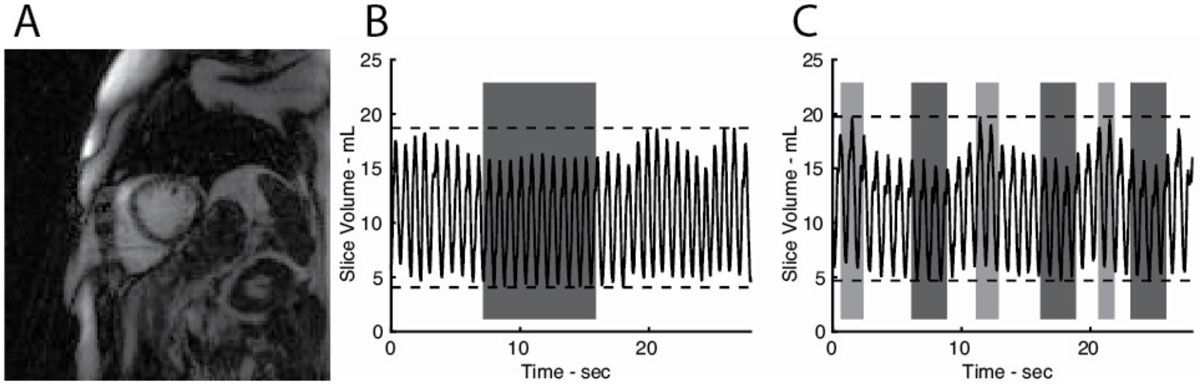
Effect of Respiratory Motion on MRI-derived Hemodynamic Measures. A Example of RT MRI Image in Short-Axis Slice. B Slice volume measured during a conventional breathhold. Gray box indicates period selected for hemodynamic measurements during breathhold. C Slice volume measured during free breathing acquisition. End-inspiration and end-expiration periods are demarcated with light and dark gray boxes, respectively.
